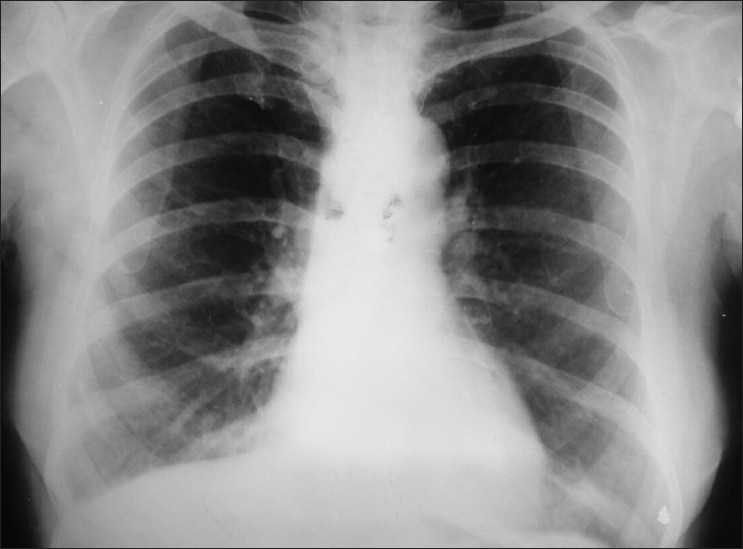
Chest radiograph after 12 months
